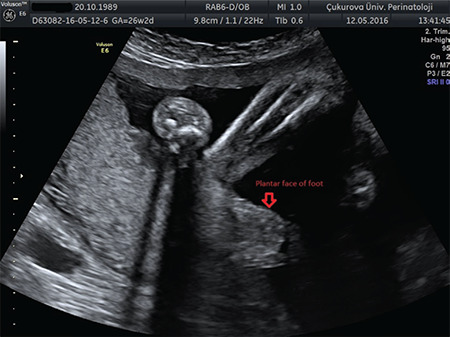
PEV ultrasonography image PEV: Pes equinovarus
